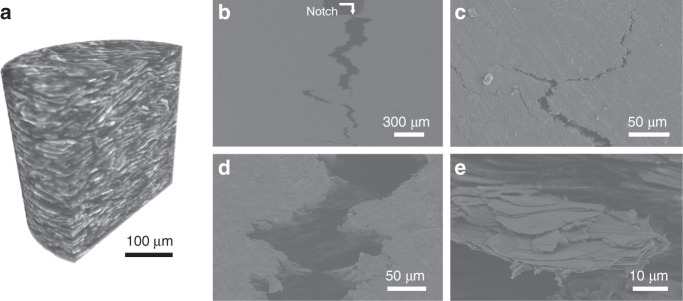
Brick-and-mortar structure and multiscale extrinsic toughening mechanisms. a 3D reconstruction of the all-natural bioinspired structural material derived from X-ray microtomography. b Fracture surface of an all-natural bioinspired structural material sample that shows long-range crack deflection. c Crack deflection, branching, bridging, and multiple cracking towards the end of the crack path. d Pulling out of TiO_2_-mica microplatelets. e Details of the fracture surface showing TiO_2_-mica microplatelet’s pulling out, delamination, CNF bridging, and stretching.
